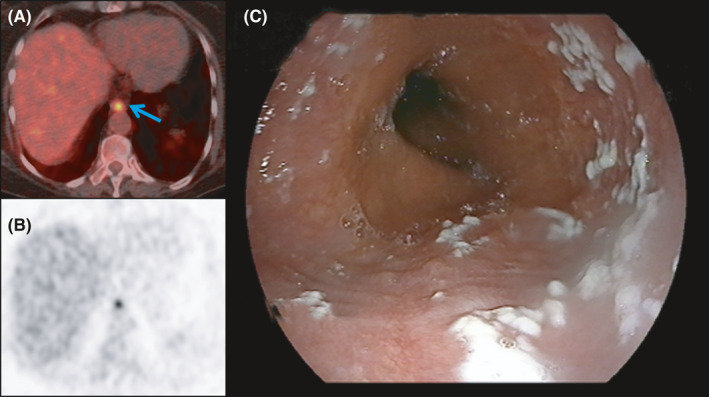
Axial 18F‐FDG PET/CT images showed focal 18F‐FDG uptake in the distal esophagus (SUVmax: 8.68), suggesting an etiology that may have clinical significance (A—solid arrow and B). Upper endoscopy demonstrated multiple whitish plaques corresponding to focal FDG uptake in the distal esophagus (C)
